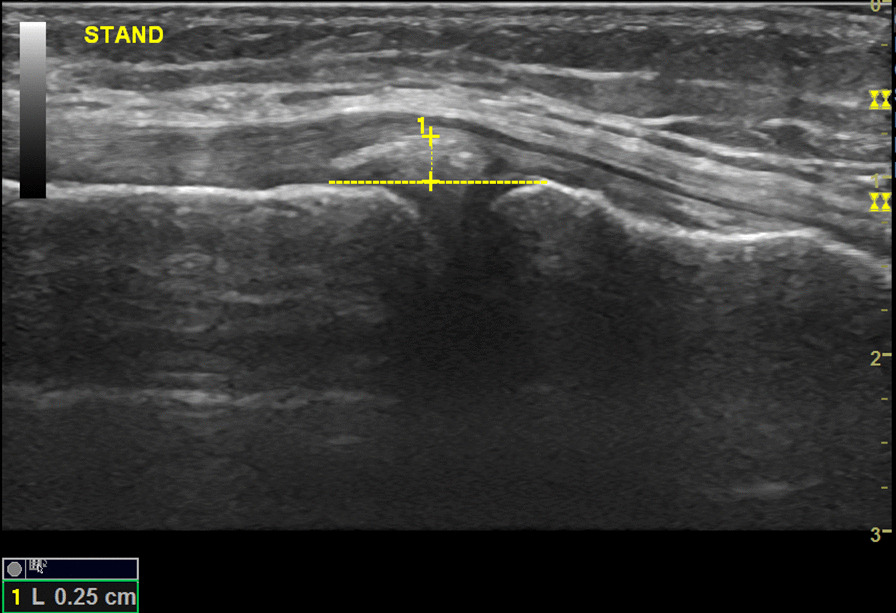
Sonography of the medial meniscus deep to the MCL while standing
